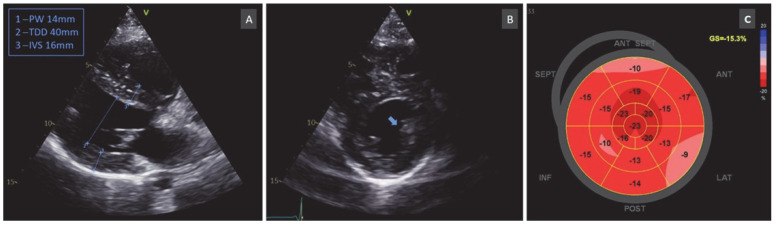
Transthoracic 2D echocardiography: (A) Parasternal long-axis section showing concentric hypertrophy involving mainly the basal septum (diastolic interventricular septum thickness of 16 mm). (B) Parasternal midventricular short axis revealing a prominent anterolateral papillary muscle (blue arrow). (C) Bull’s eye of LV myocardial deformation showing abnormal values of a global longitudinal strain of −5.3%.
